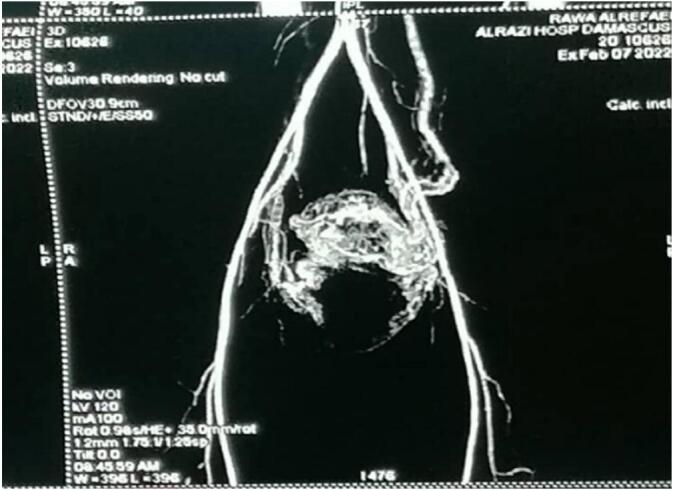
Bilateral arterial and venous uterine fistulas from the bilateral internal iliac arteries by computed tomography.
